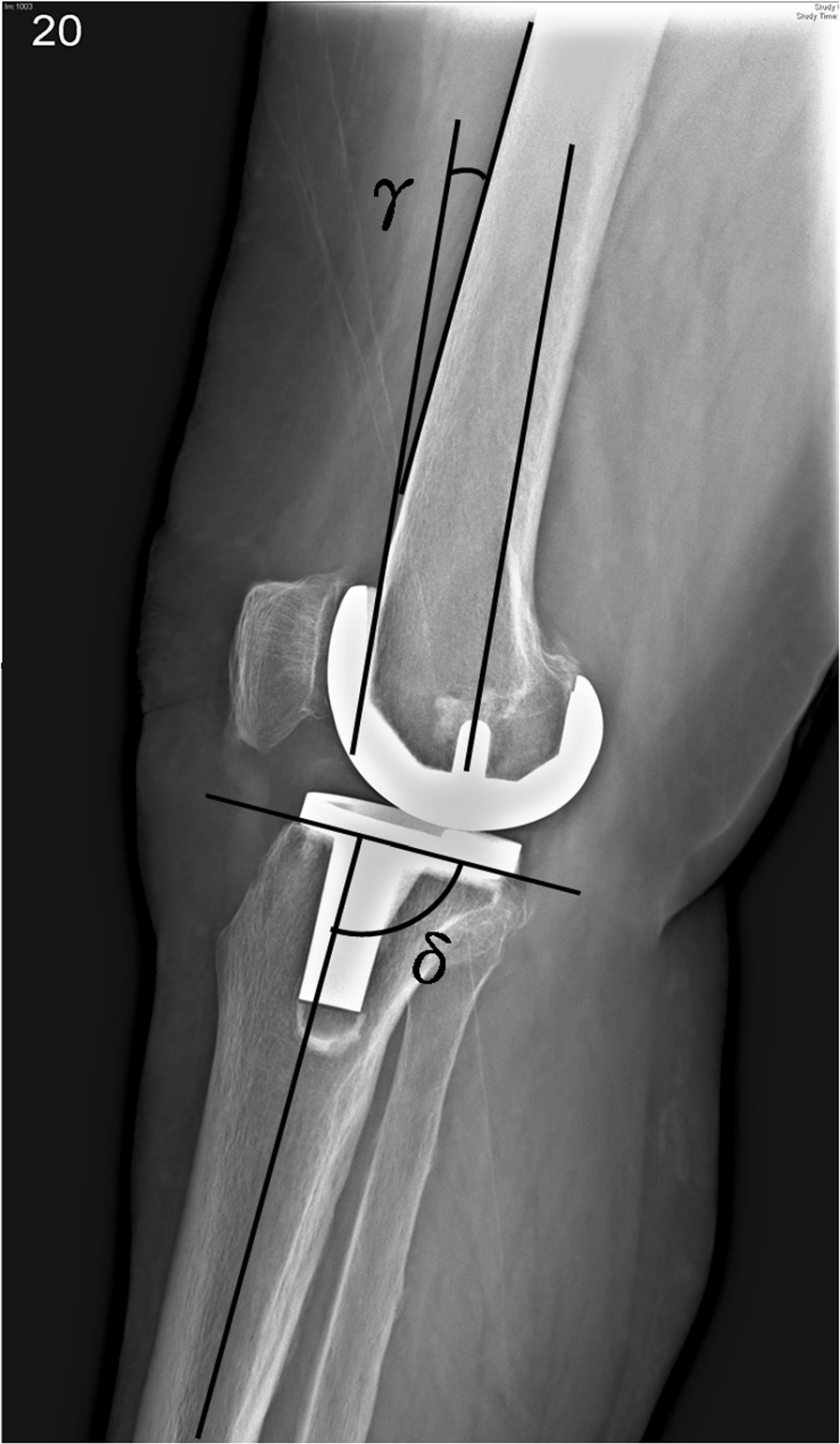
Radiograph showing the measurement of flexion and extension of the femoral component and measurement of the posterior slope of the tibial component in the sagittal plane. Radiographic evaluation system originally appeared in J Bone Joint Surg Am. 2009;91:14–19. *γ* sagittal femoral angle, *δ* sagittal tibial angle
